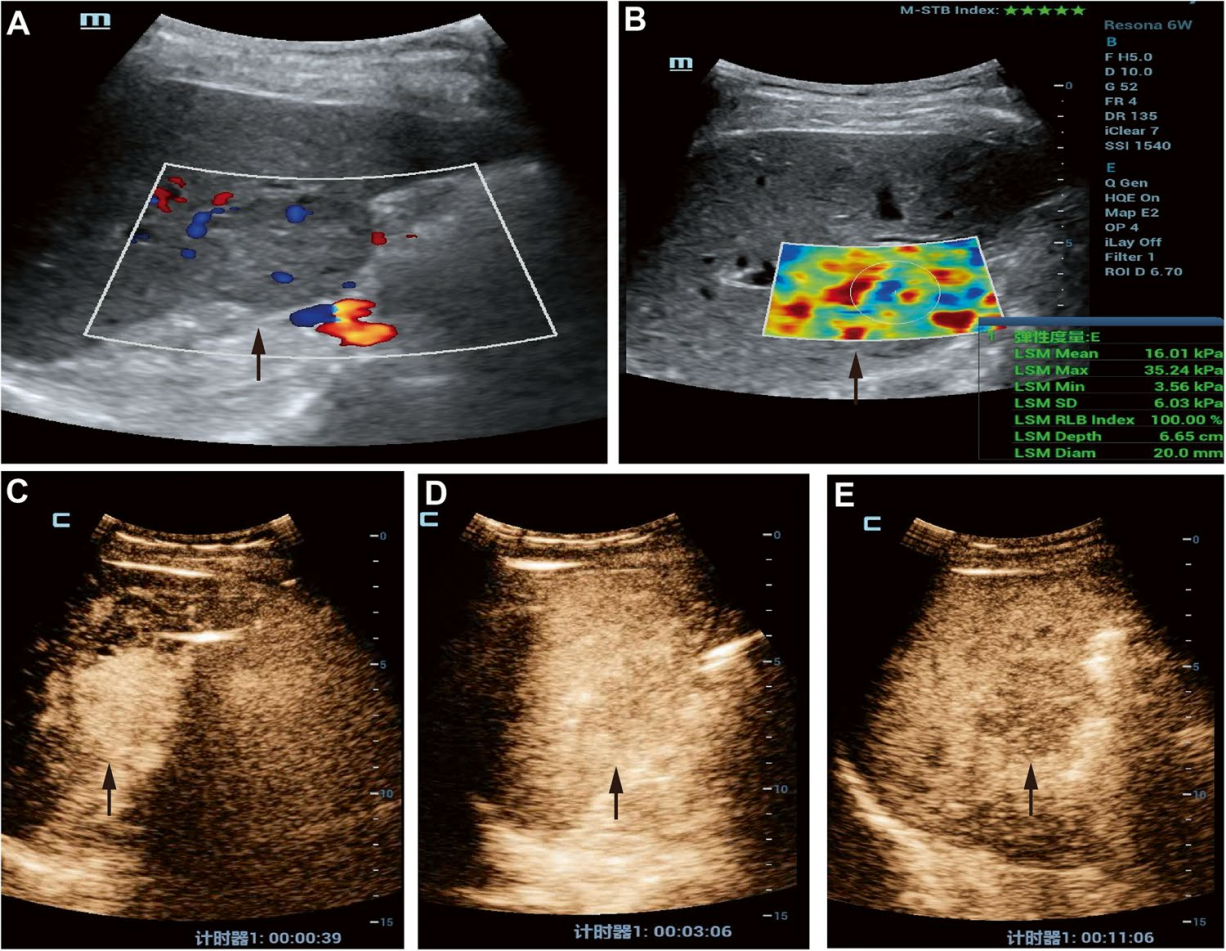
A 33-year-old HCC patient with an ALT of 23.4U/L. (A) Grayscale ultrasound shows hypoechoic lesions in the liver, with abundant blood flow and a maximum diameter of approximately 3.3 cm. Clear edges and regular shapes; (B) The STE display shows Emean as 16.01 kPa; (C) APHE appeared in the arterial phase after injection of Sonazoid contrast agent. (D) After 3 min, the lesion had no obvious washout; (E) After 10 min (Kupffer phase), the lesion showed low enhancement. According to CEUS LI-RADS, the lesion was considered benign. According to the column chart, the risk value is greater than 0.8); The lesion is predicted as malignant. Pathological diagnosis of highly differentiated HCC.
